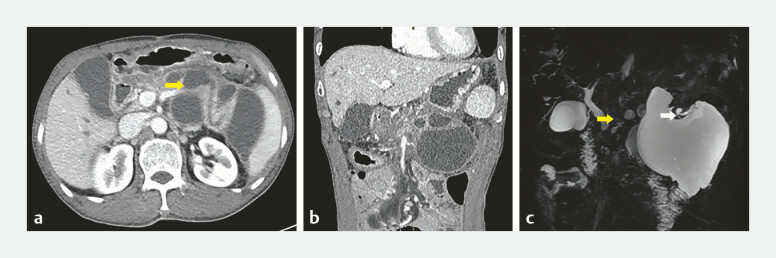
Preoperative abdominal CT and MRCP.
